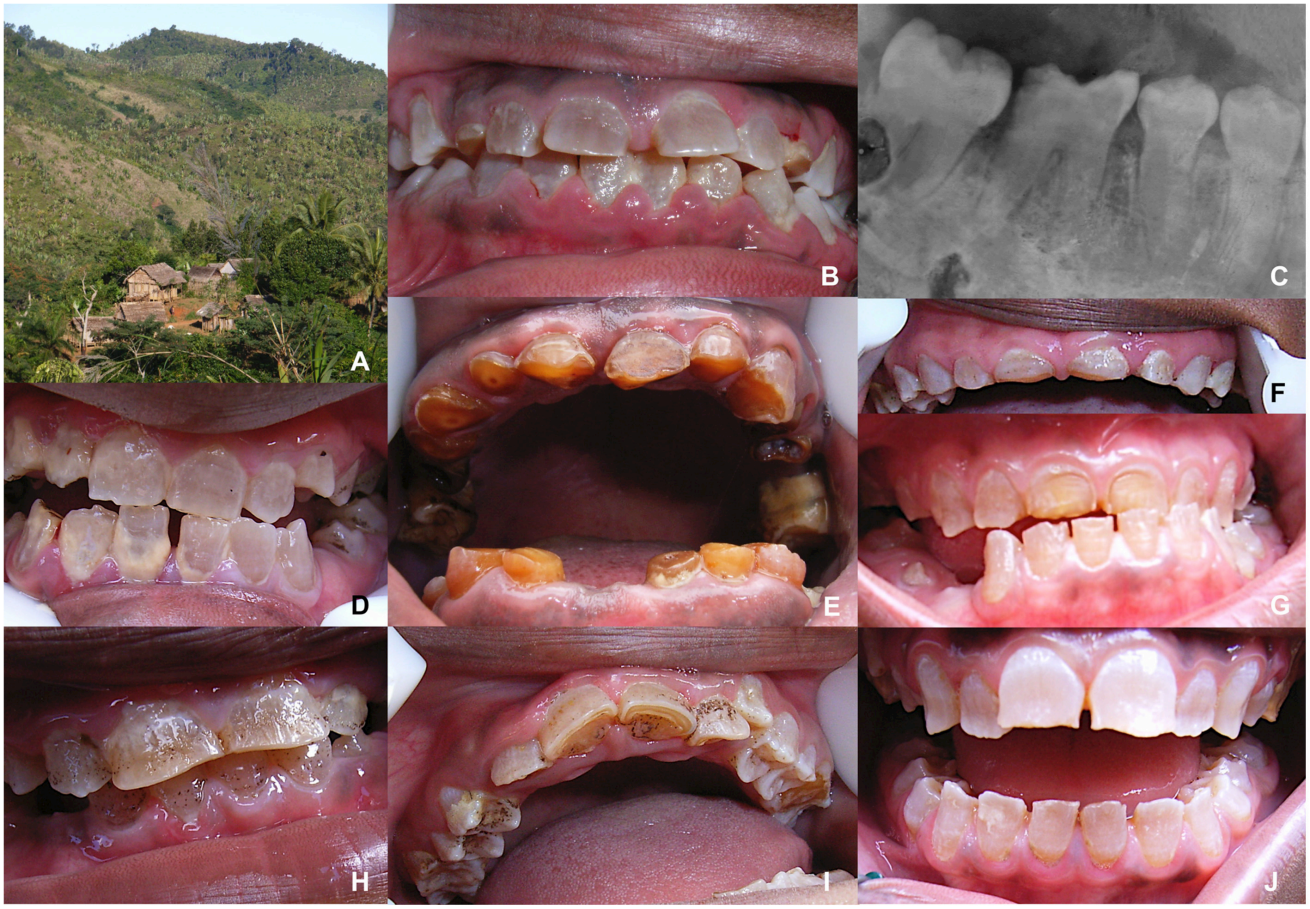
Clinical description of the disease. (A) The remote village of Antananarivo. (B–J) Inhabitants'dentition showing the typical features of dentinogenesis imperfecta with the gray-brown discolouration of the dentin clearly visible after enamel cleavage and progressive tooth wear. (C) On the retro-alveolar radiography of the lower right premolar/molar sector of individual (B), cervical constriction, short roots and the disappearance of pulp spaces due to erratic dentin formation represent the characteristic hallmarks of dentinogenesis imperfecta. (H–J) In addition to dentin anomalies, hypoplastic enamel defects exist, with the presence of pits, striae and flattened buccal surfaces.
